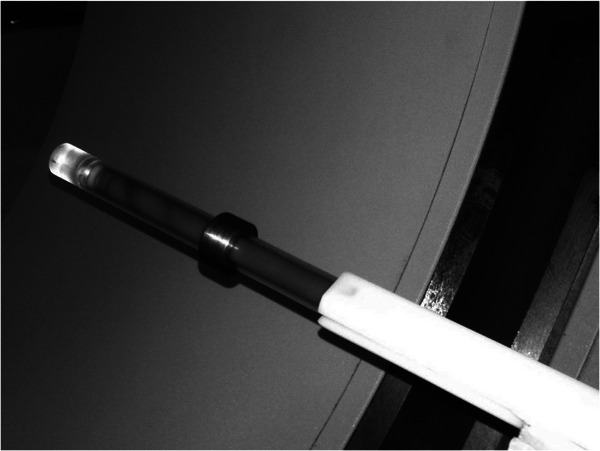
Pencil ionization chamber with tungsten mask positioned CT scanner gantry.
